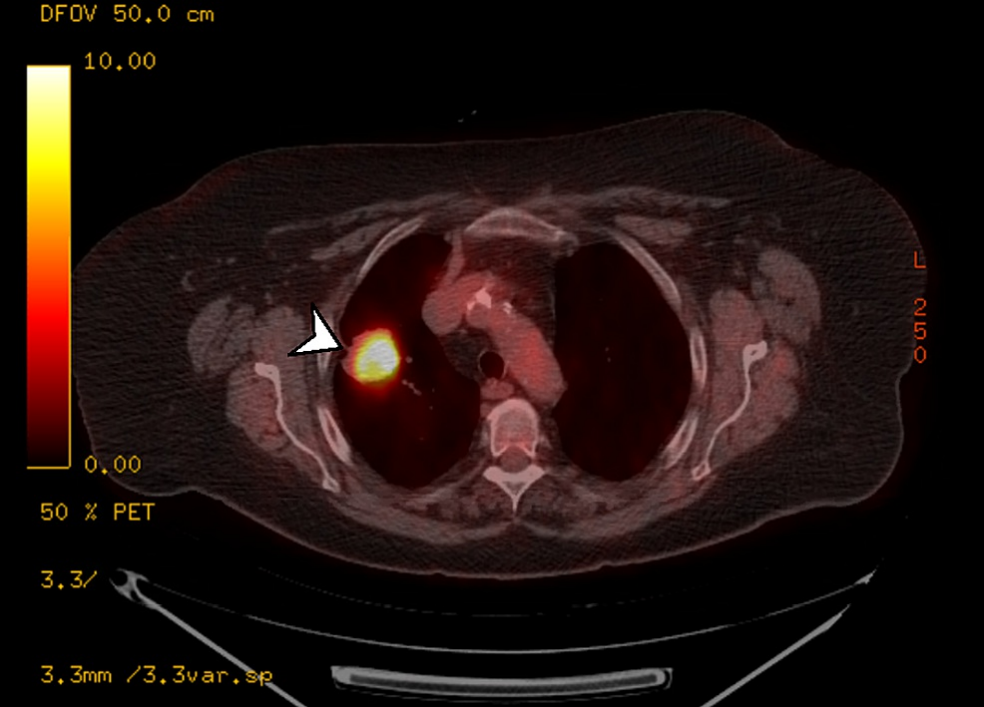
PET-CT showing the hypermetabolic state of the nodule (white arrowhead) PET-CT: positron emission tomography-computed tomography
